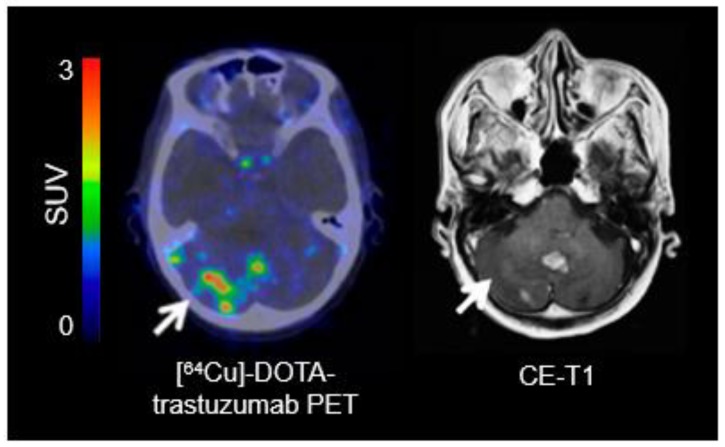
[^64^Cu]-DOTA-trastuzumab positron-emission tomography (PET) and contrast-enhanced magnetic resonance imaging (MRI) performed one day after initiation of treatment with trastuzumab in a patient with a human epidermal growth factor receptor 2 (HER2)-positive breast cancer with brain metastases. In single brain metastases, [^64^Cu]-DOTA-trastuzumab PET helps to improve lesion detection (arrow) (modified from Tamura et al. [160], with permission from the Society of Nuclear Medicine and Molecular Imaging).
